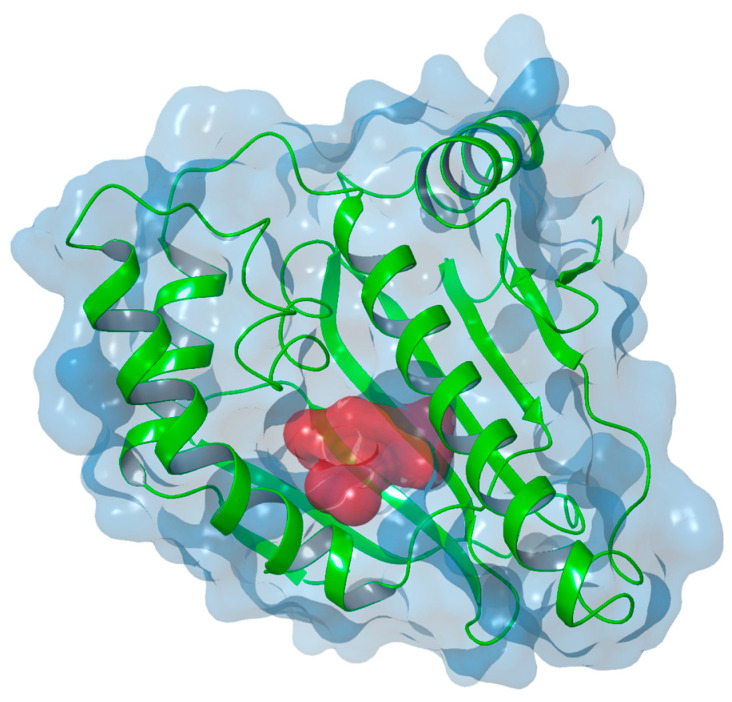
X-ray structure of the Hsp90 ligand binding pocket (PDB code 2FWY [42]).
